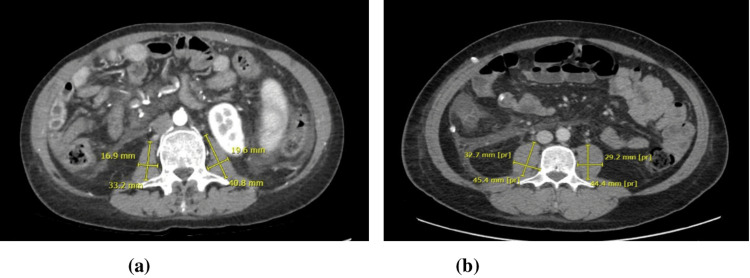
Psoas major muscle assessment images in a liver transplant patient. (a) Abdominal CT image (horizontal section) showing the third lumbar vertebra to calculate the PMI for pre-therapeutic exercises. (b) Abdominal CT image (horizontal section) showing the third lumbar vertebra to calculate the PMI for post-therapeutic exercises. CT: computed tomography; PMI: psoas muscle index
